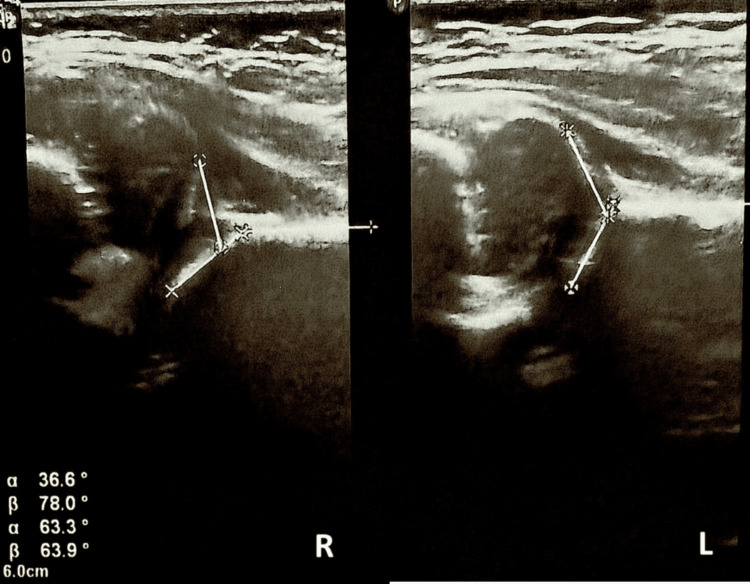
Graf ultrasound measurements demonstrating type IV hip dysplasia with a decreased alpha angle and an increased beta angle on the right side, on the left side the angles are normal. R: right. L: left
